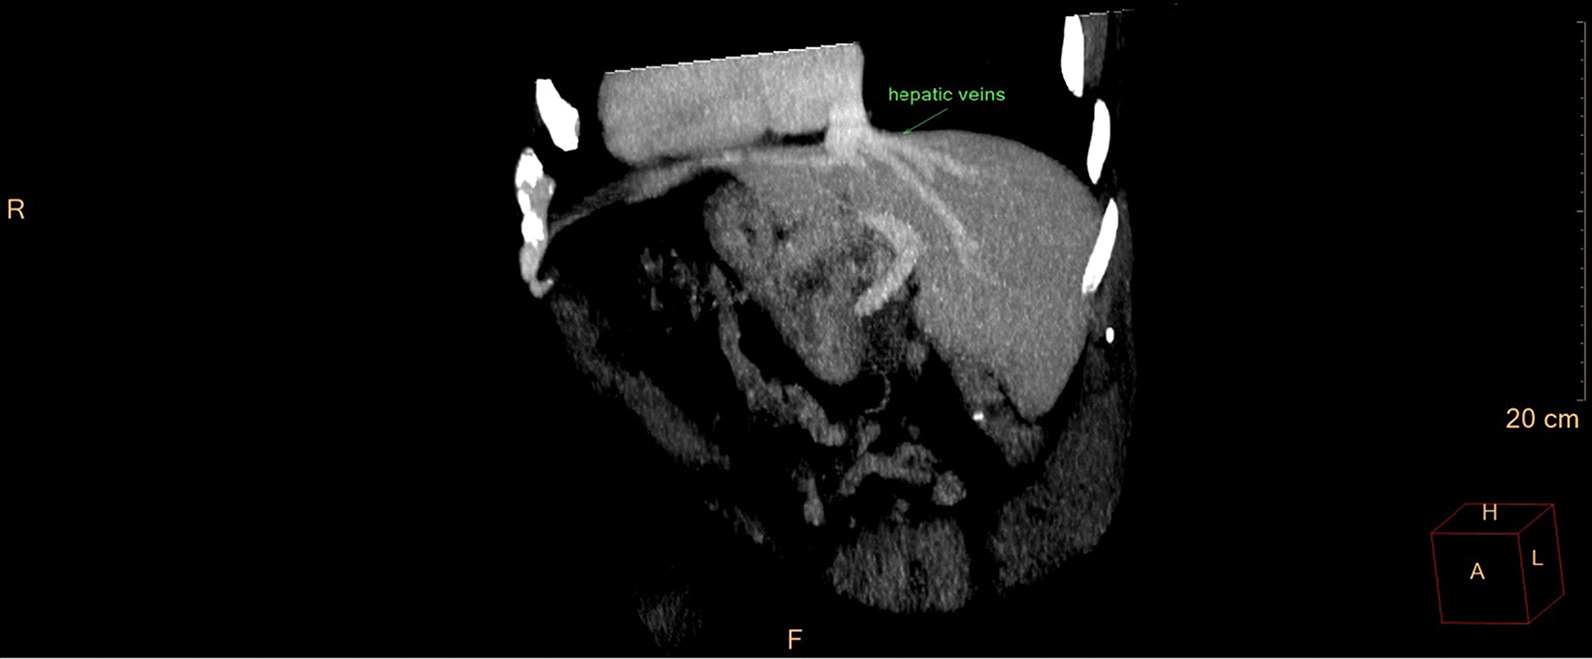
CT—maximum intensity projection—all hepatic veins drain directly into the atrium
